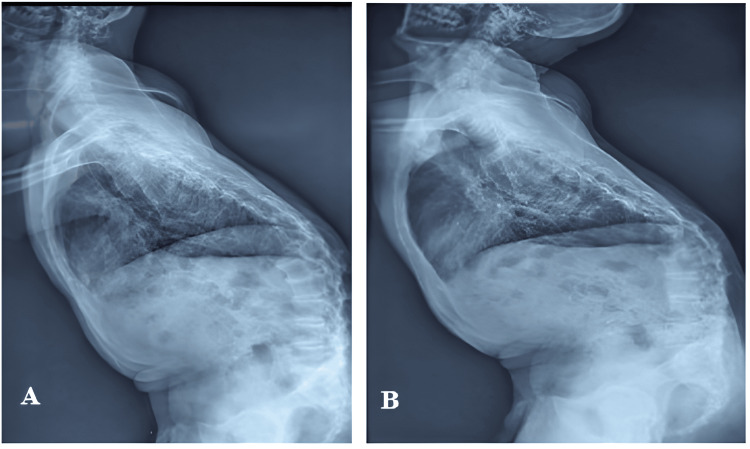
Lateral standing spine radiographs demonstrating severe thoracolumbar kyphosis in advanced ankylosing spondylitis. (A) Without postural correction. (B) Attempted postural correction showing minimal improvement, consistent with a fixed deformity.
